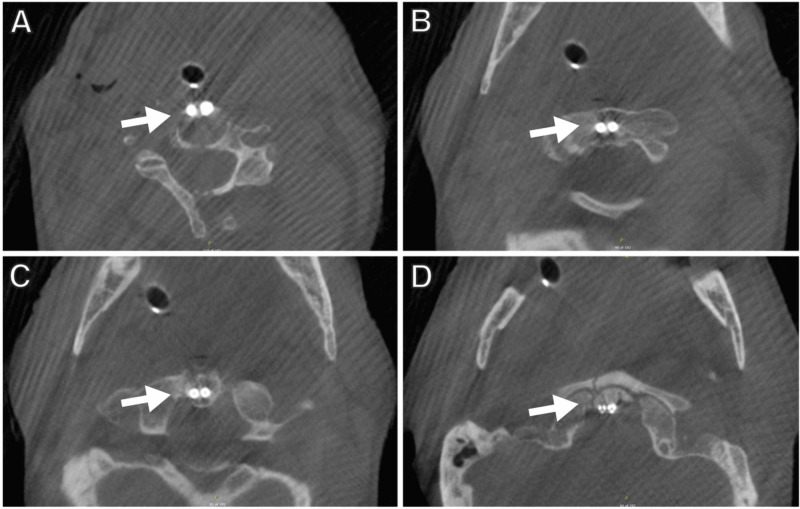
Final intraoperative images The final CT spine confirms placement of the odontoid screws (indicated with arrows) from (A) the anterior endplate of C2, traversing the (B) C2 body and (C) base of the odontoid process, and (D) penetrating the cortex of the odontoid tip CT: computed tomography
